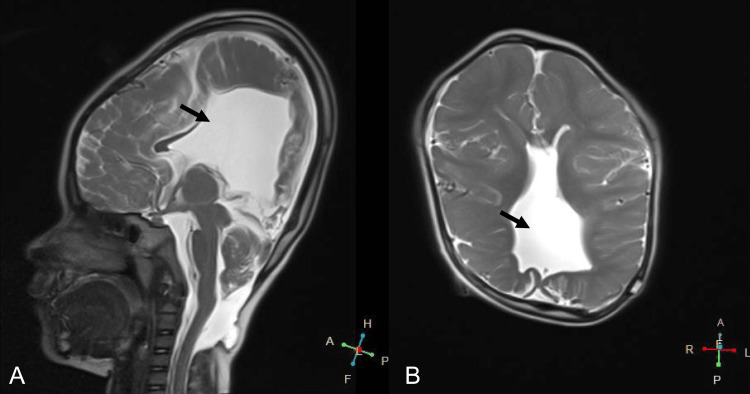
MRI T2 imaging at the age of four A) Sagittal view, the black arrow highlights the ventricle remaining reduced in size; B) Axial view, the black arrow highlights the ventricle remaining reduced in size MRI, magnetic resonance imaging
